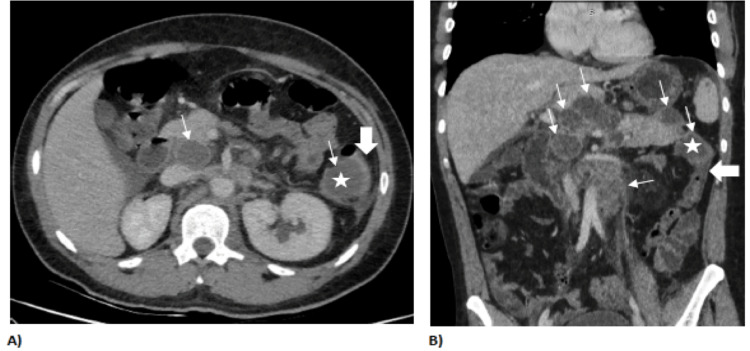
Multiple necrotic abdominal and retroperitoneal lymphadenopathy on abdominal CT with IV contrast. Axial (A) and coronal (B) study before IV contrast, before colonoscopy, showing multiple retroperitoneal necrotic lymphadenopathy (thin white arrows), with one of them (asterisk) intimately related to the most proximal descending colon (thick white arrow). IV, intravenous
